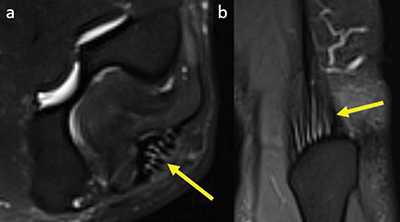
Axial (a) and coronal (b) fat-saturated T2-weighted image at the level of the triceps tendon insertion with a physiological linear T2-hyperintense signal compared to muscle parallel to tendon fibers, score 2.
